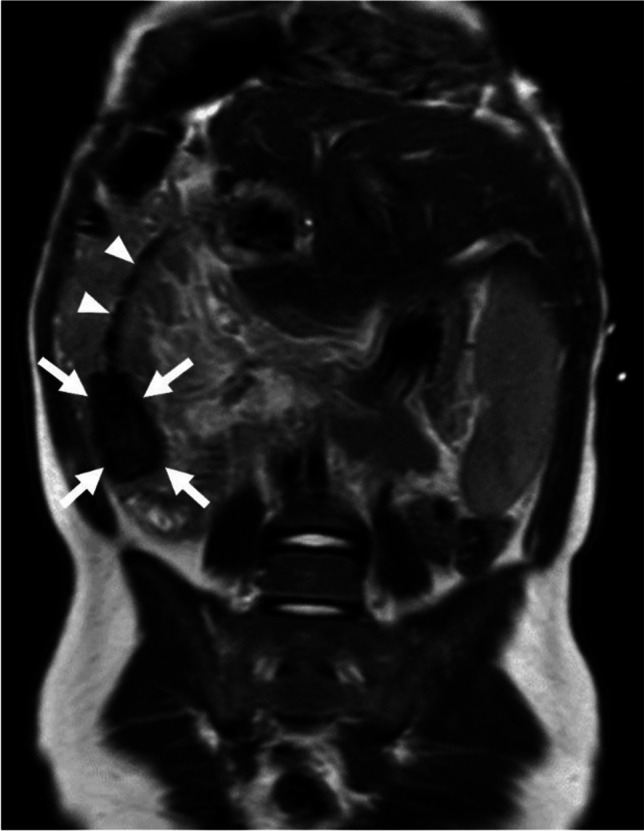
Abdominal magnetic resonance imaging of a 5-year-old girl with a percutaneous transhepatic biliary drainage after liver transplantation. Coronal T2-weighted image shows a hypointense structure (*arrows*) measuring 1.6 × 1.6 × 3.5 cm at the distal end of the biliary drainage (*arrowheads*) in the bowel lumen in the right lower quadrant
